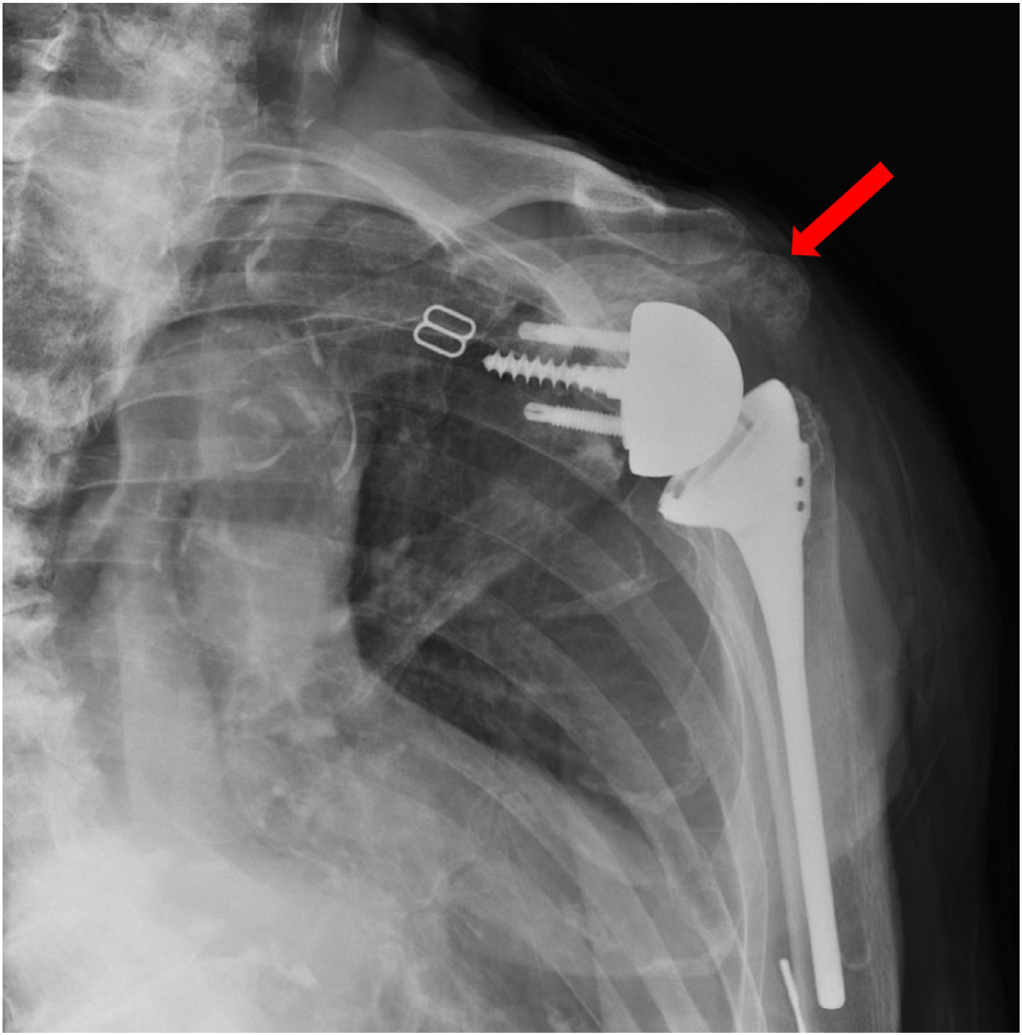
X-ray Grashey view demonstrating an acromial fracture with inferior tilt.
